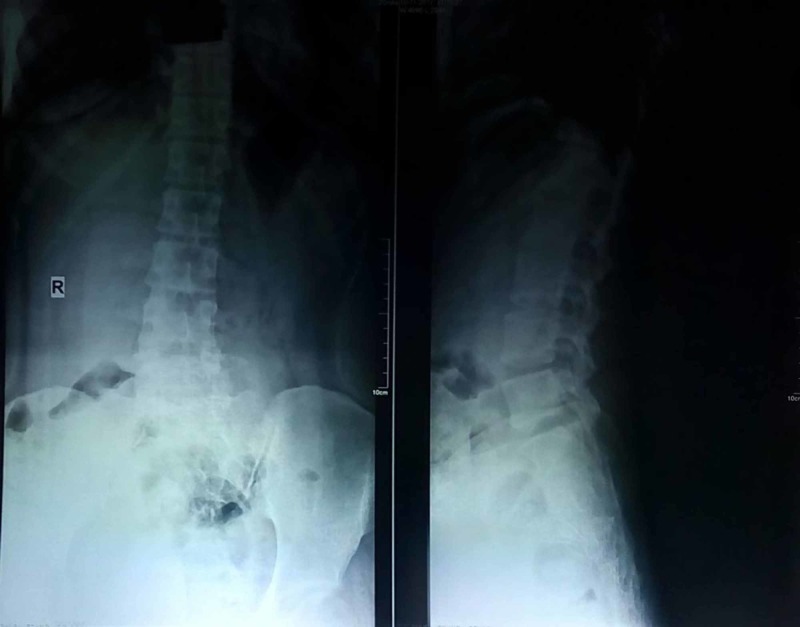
Three-year follow-up radiograph shows no evidence of recurrence or reappearance of the sacral lesion
